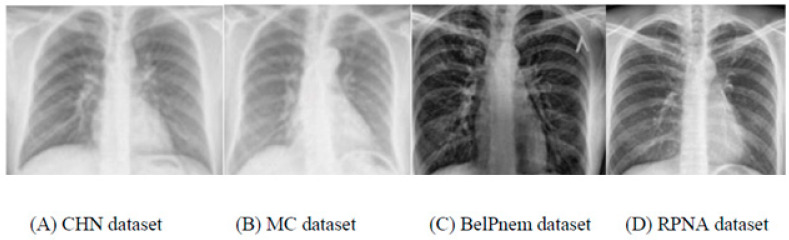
Example of X-rays from the utilized databases. (A) CHN dataset, (B) MC dataset, (C) BelPnem dataset and (D) RPNA dataset.
